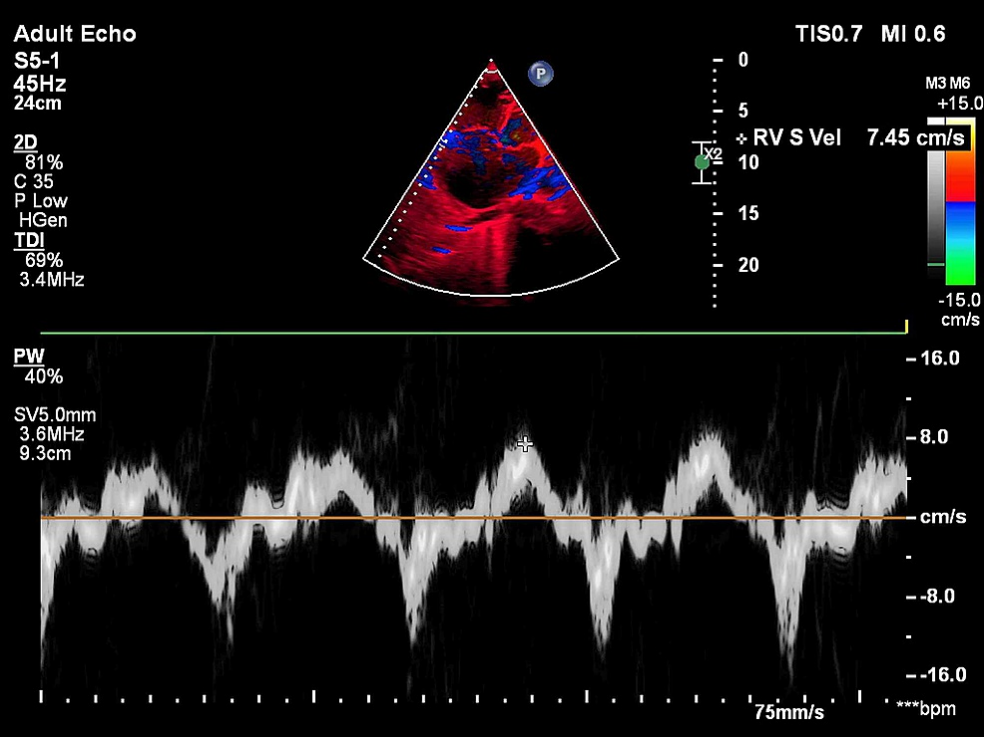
Tissue Doppler imaging showing right ventricle systolic excursion (RV S') velocity
